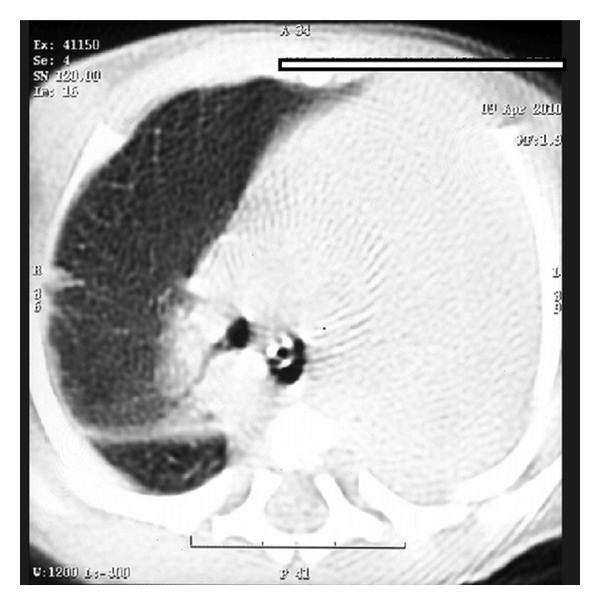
CT chest with atretic left hemithorax.
